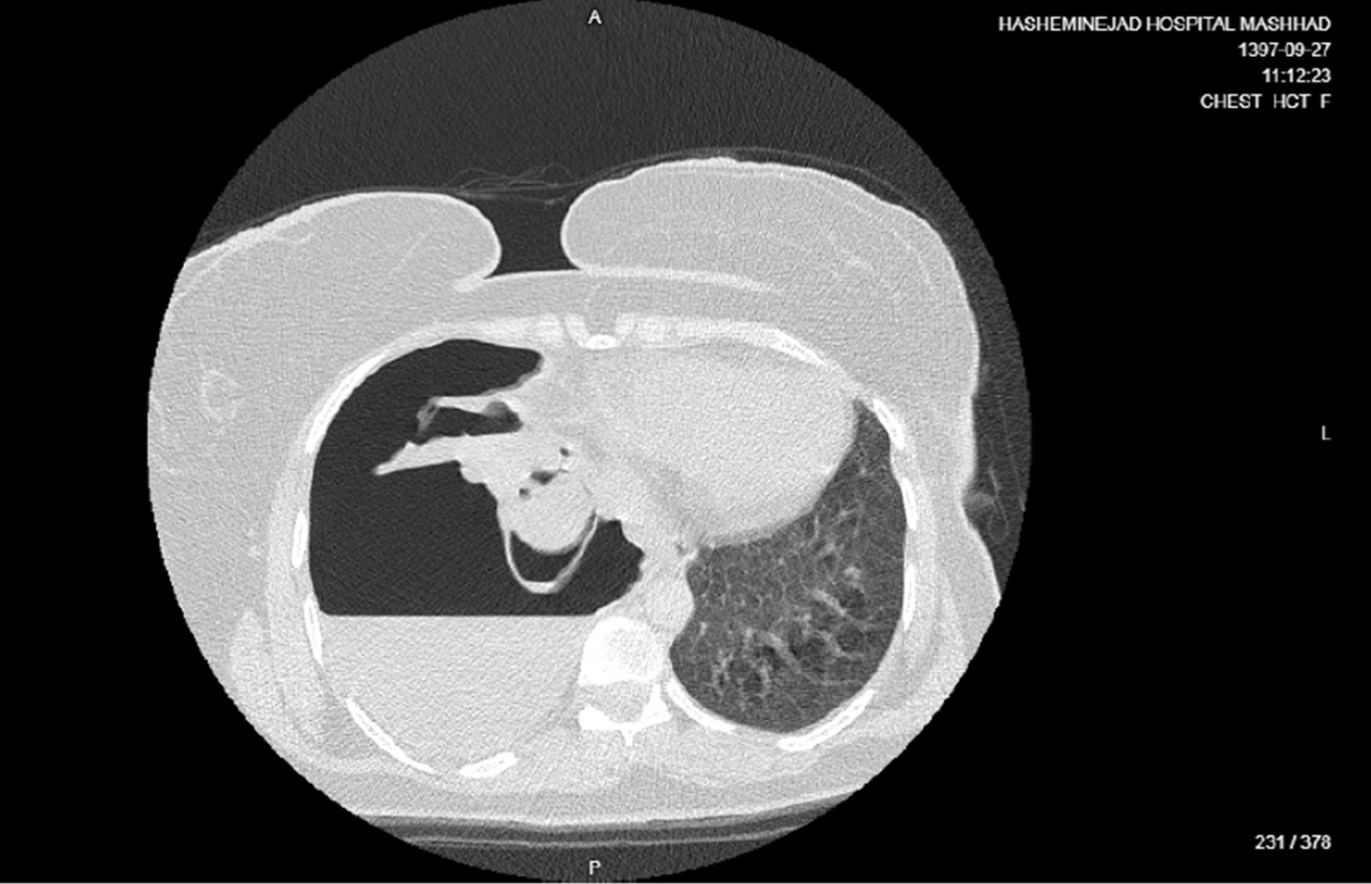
Chest CT scan of Case 4 revealed a collapsed right lung, mediastinal shift to the left side, and a cystic lesion resembling a hydatid cyst with a ruptured membrane.
